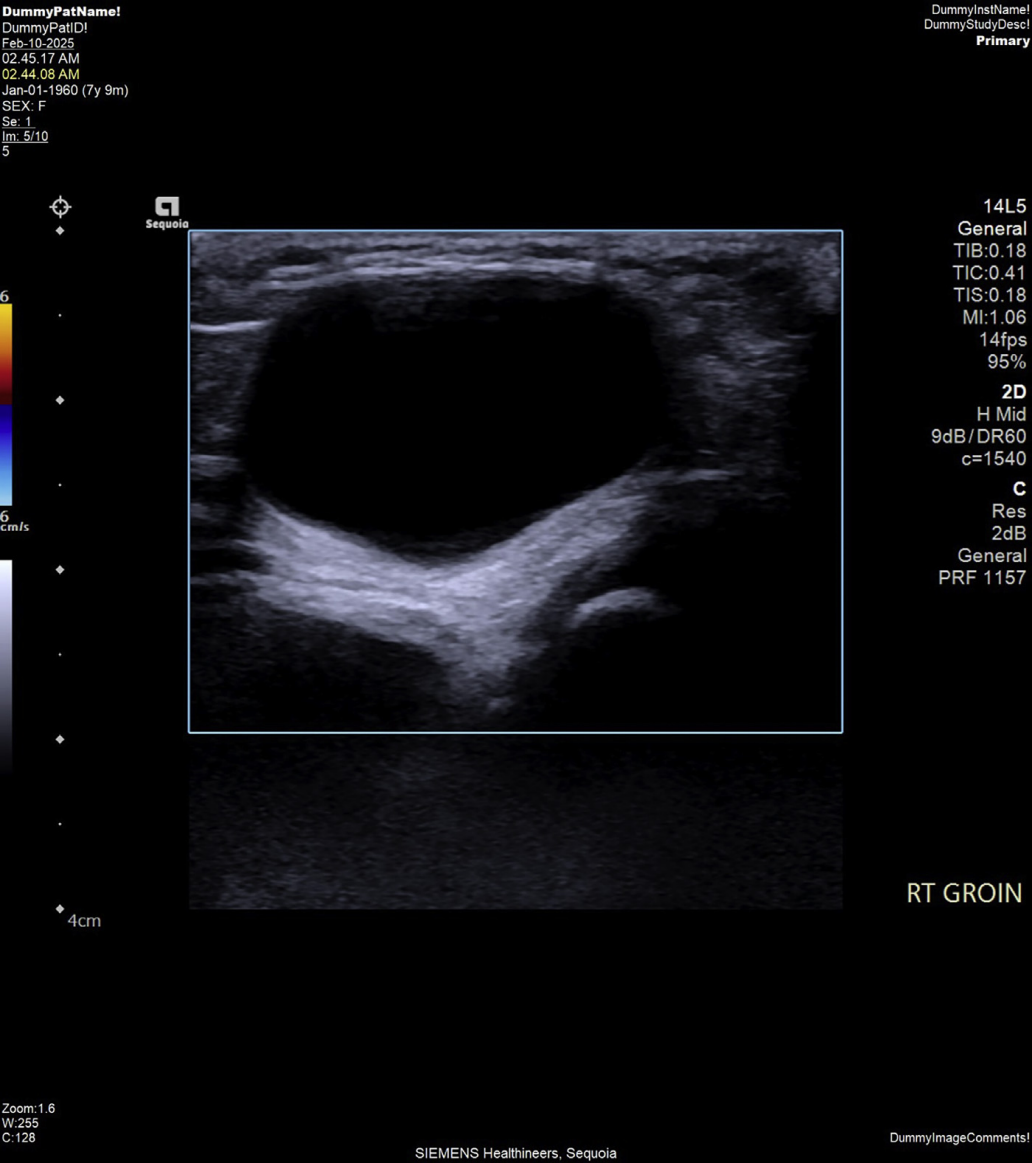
Color Doppler ultrasound image of the right groin showing a fluid-filled cystic lesion with no notable vascular uptake.
